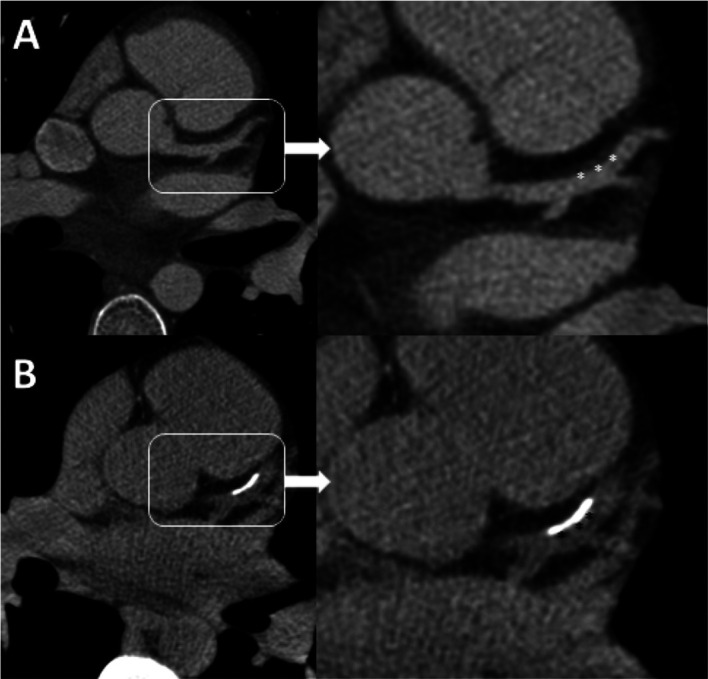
Progression of plaque. A) Initial coronary CT demonstrates subtle soft plaque/vessel wall thickening (white asterisks) in the proximal LAD. B) Calcium score, 3 years later, now shows new overlying calcification (black asterisks) implying mineralization secondary to chronic low-grade inflammation. Statin therapy should be considered for all KD patients although the level of evidence for treatment is weak.
